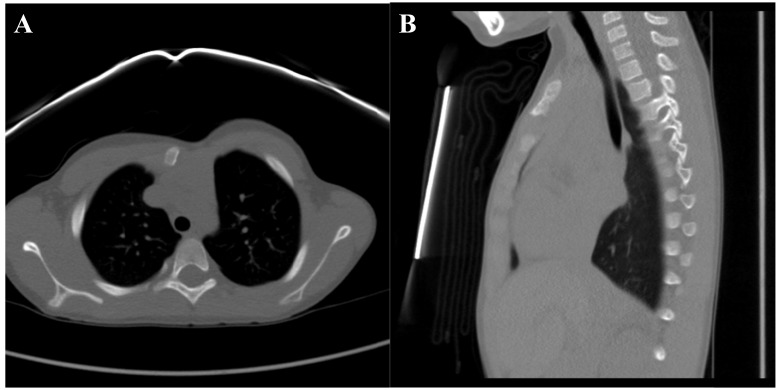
Follow up CT scan of the chest including axial Fig. (7A) and a sagittal Fig. (7B) views.
